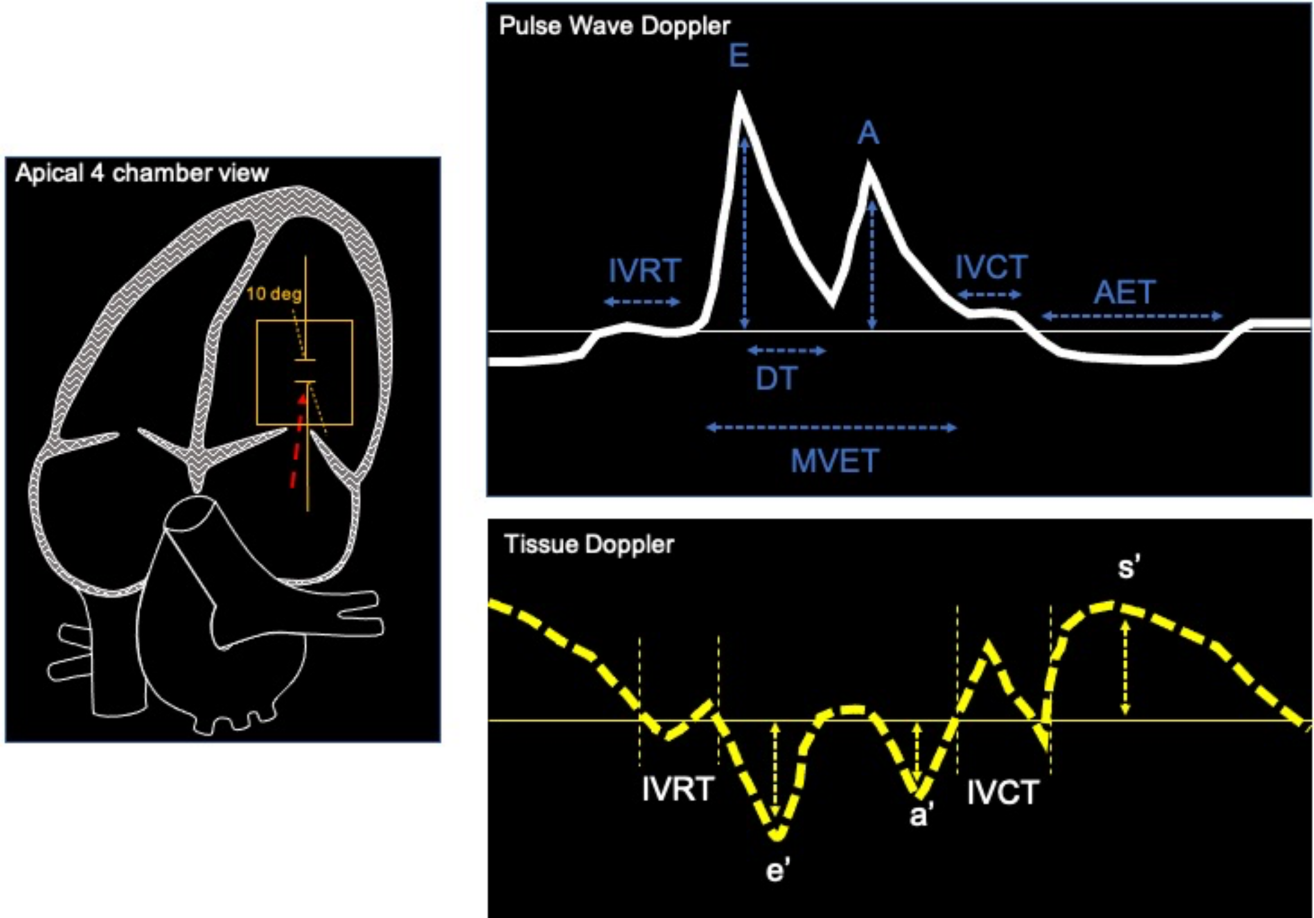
Example of apical 4 chamber view of the heart (left) used to record pulse wave Doppler (top) and tissue Doppler (bottom). Typical measurements of mitral blood flow with PW Doppler are: interventricular relaxation time, early and late mitral velocities (E and A waves), E wave deceleration time (DT), isovolumic contraction time (IVCT), aortic ejection time (AET). Tissue Doppler imaging are used to measure peak systolic velocities (s’), Peak early diastolic mitral annular velocities (e’) during early filling at septal or lateral corner of mitral annulus, Peak velocity in atrial systole (a’).
